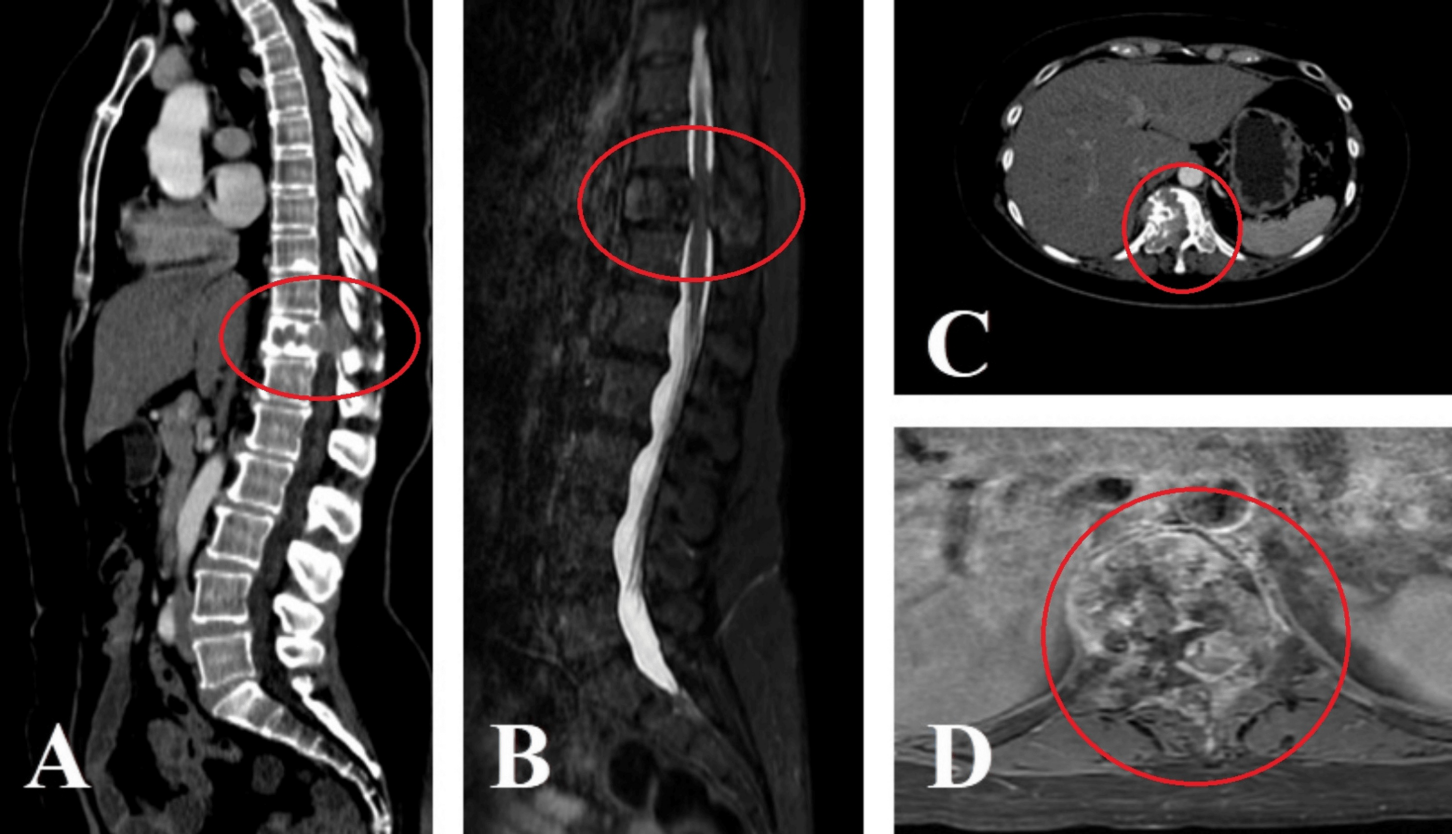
(A) Computed tomography (CT) scan of the spine shows a T11 pathological compression fracture with displacement of the posterior wall and invasion of the spinal canal. (B) MRI scan of the spine shows compression of the spinal canal due to a metastatic lesion. (C and D) CT and MRI axial sections show a lytic lesion of the vertebral body of T11, facets, and right pedicle, as well as the lamina and spinous process.
